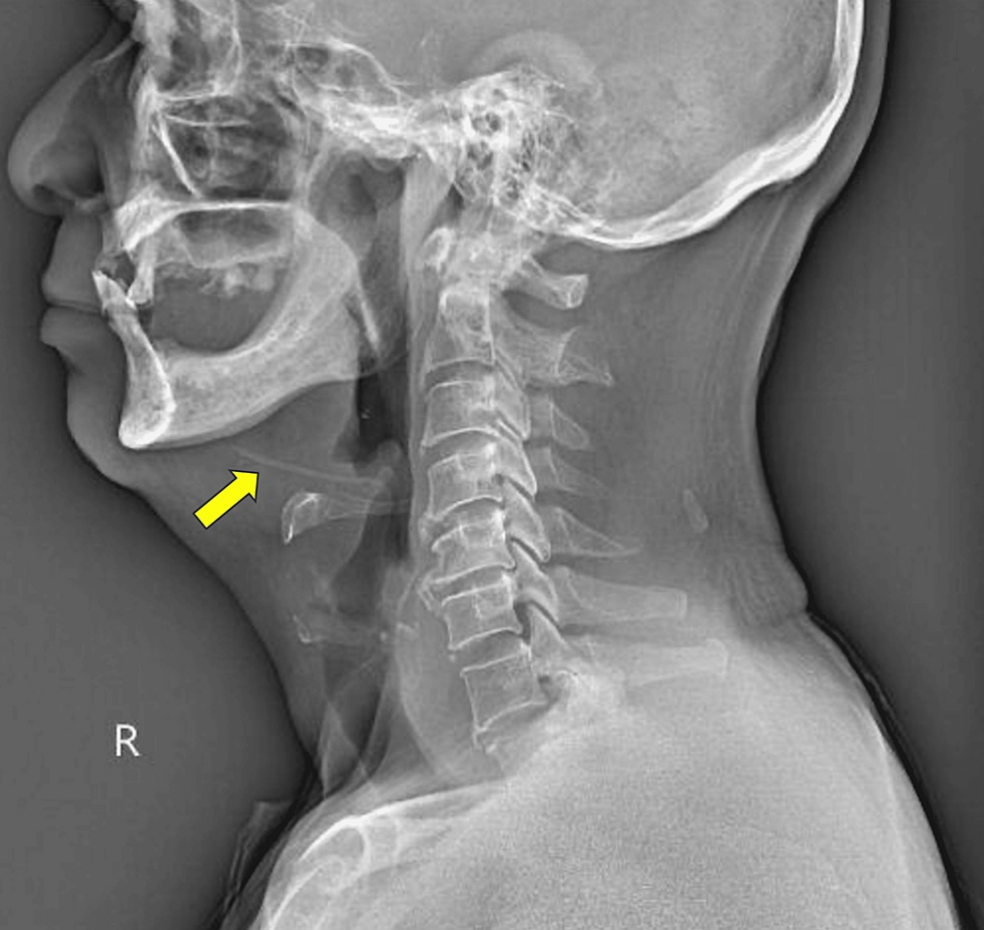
Lateral neck radiograph revealing a linear opacity suggestive of a foreign body within the anterior soft tissue of the neck at the level of the third cervical vertebra (yellow arrow).
